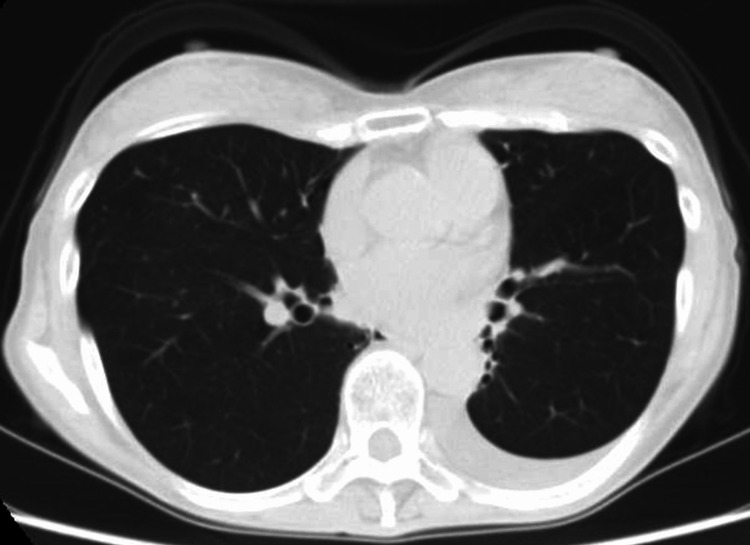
A computed tomography scan of the bronchi A computed tomography scan demonstrating that the bronchi in the left lung are tractioned toward the mediastinum, thus indicating decreased lung volume.
